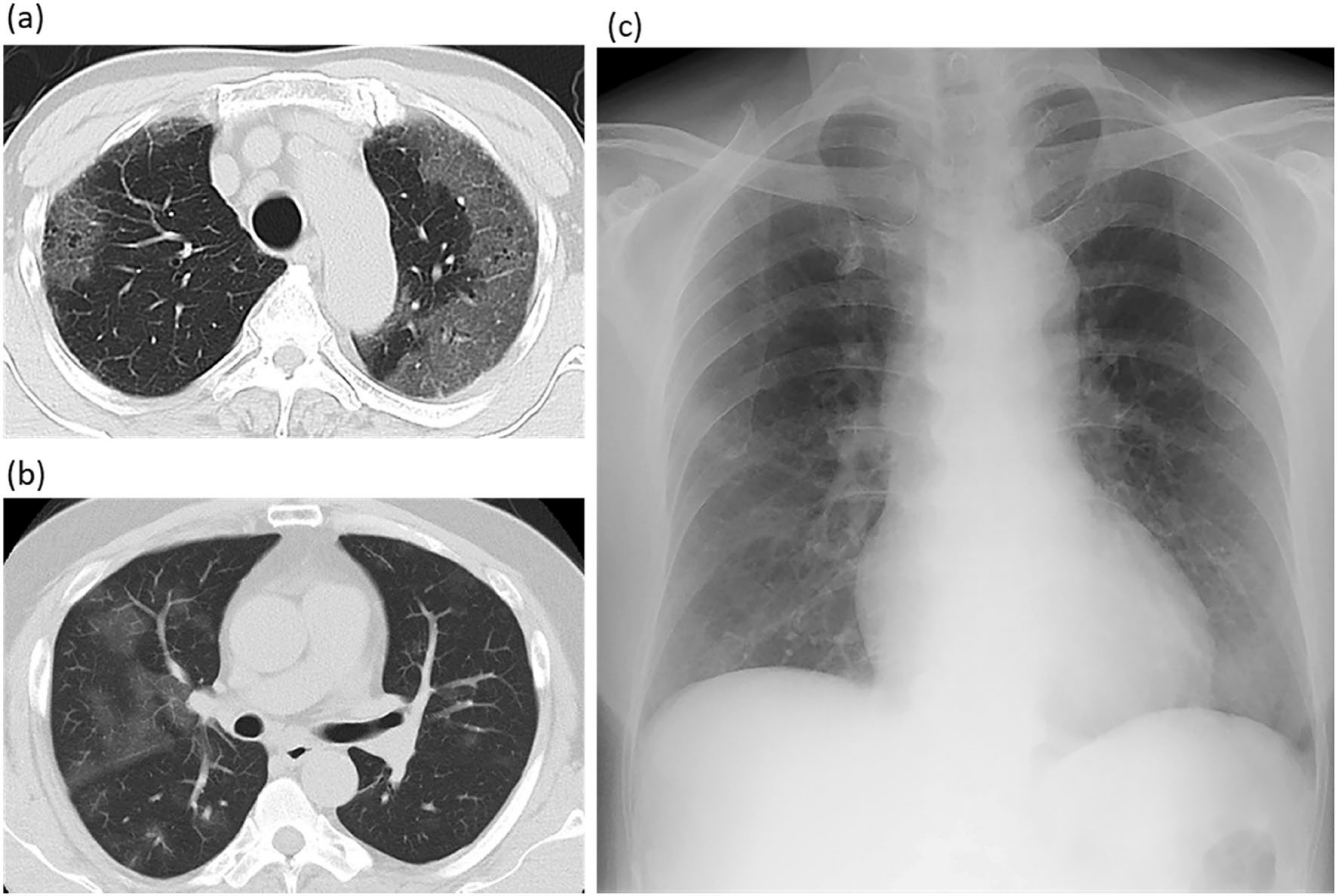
COVID-19 chest CT and X-ray images on admission. a Chest CT image of a 68-year-old male patient with ground-glass opacities (GGO) in the peripheral region (non-severe case). b Chest CT image of a 51-year-old male patient with GGO in the peripheral and central regions (non-severe case). c Anterior–posterior (AP) chest X-ray image of a 70-year-old female patient with cardiomegaly (severe case)
